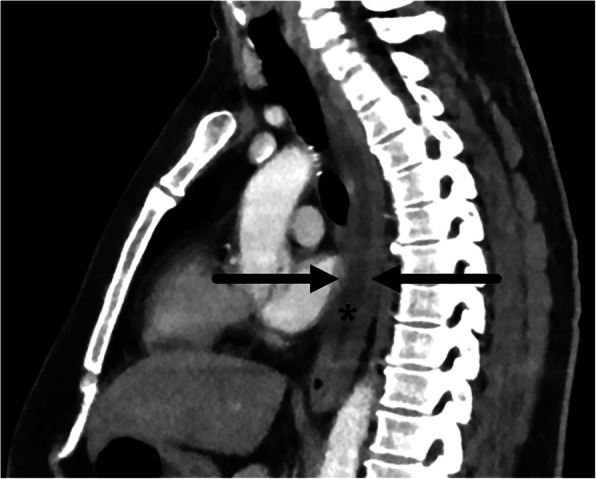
Acute esophagitis. Fifty-year-old male with diffuse chest pain and mild fever. Sagittal CT image show diffuse circumferential wall thickening with mild enhancement involving almost entire esophagus (arrows). Intraluminal fluid (asterisk) is noted
